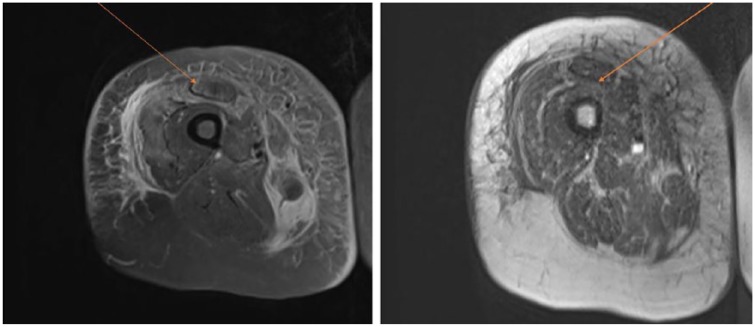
Magnetic resonance imaging showing notable soft tissue edema/inflammation in the right thigh primarily involving the anterior compartment, most advanced in the rectus femoris muscle, to lesser extent the vastus musculature, sartorius, and gracilis. There is suspected hemorrhage within the proximal right rectus femoris muscle but no organized fluid collection/hematoma (arrows).
